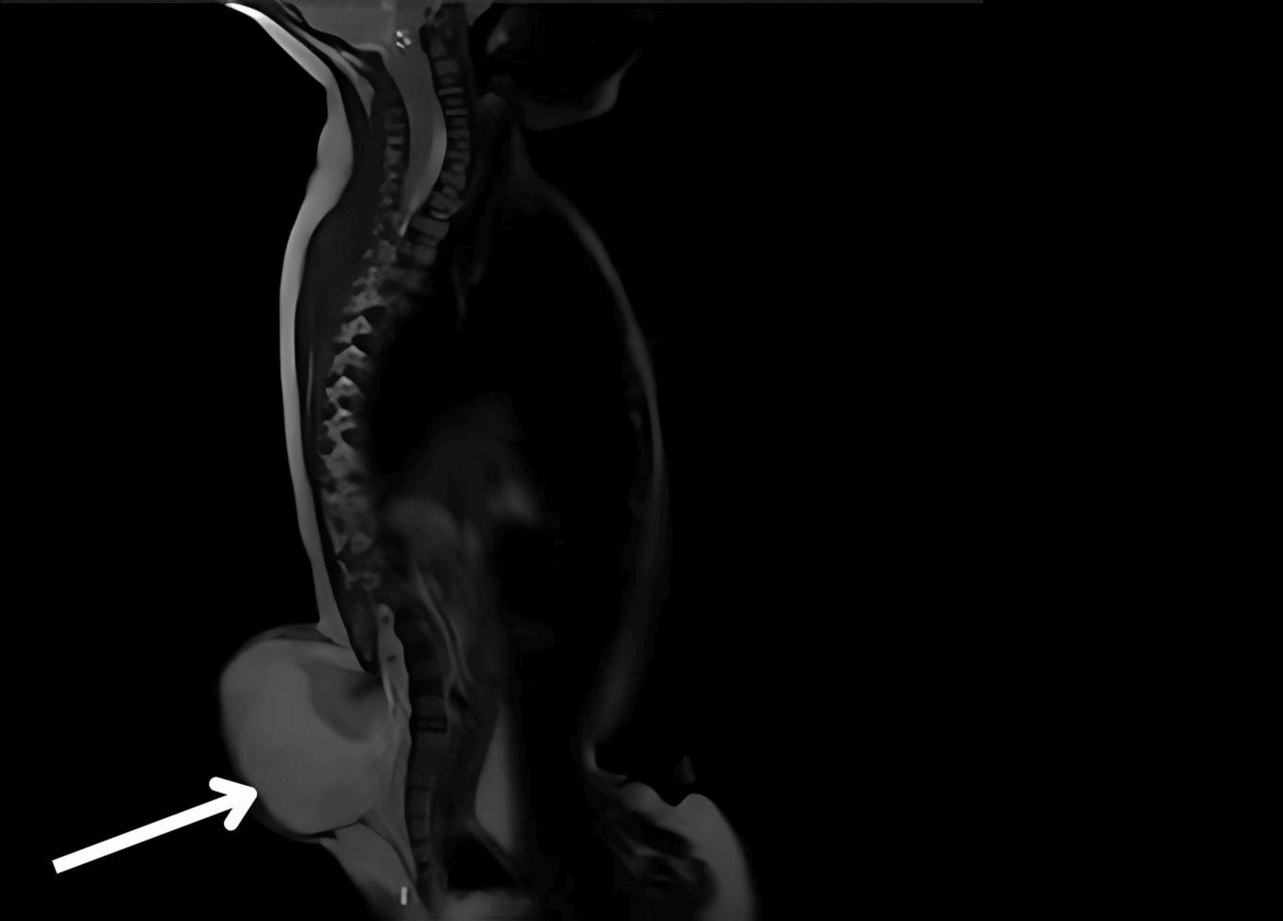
MRI sagittal T2-weighted sequence of the spine in Type 2 Chiari malformation showing absence of the posterior element at the L4 vertebral level with herniation and formation of a fluid-filled sac containing cerebrospinal fluid and neural elements (white arrow). These features are suggestive of myelomeningocele.
